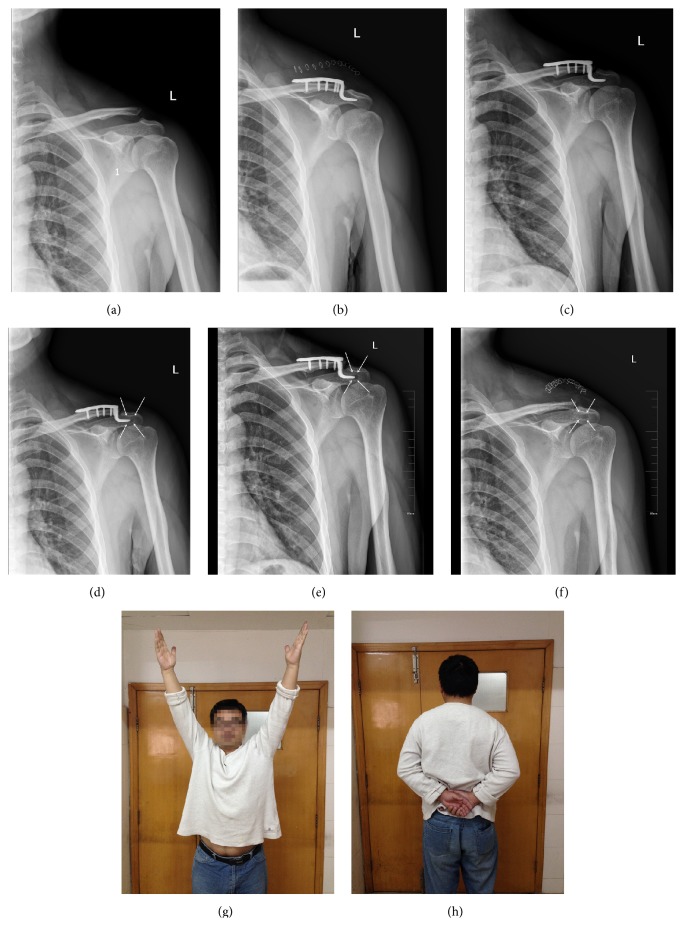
A 30-year-old male patient was diagnosed with left Degree-III acromioclavicular joint dislocation (a) and underwent surgery with a clavicle hook plate (b). The figures show his radiography review two months after surgery (c) and the occurrence of typical subacromial osteolysis nearly five months later (d). The plate was removed at the 10th month after surgery (e-f), and the patient was satisfied with his postoperative shoulder function (g-h).
